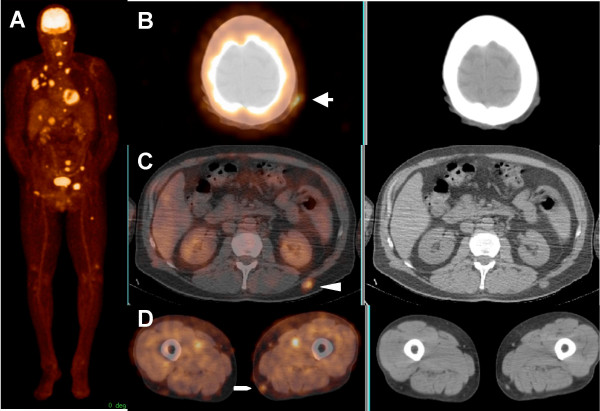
71-year-old male with history of melanoma in the right anterior chest, status post surgical resection and interleukin-2 therapy 2 weeks prior to the PET/CT scan. Maximum intensity projection (MIP) image (A) and transaxial images (B, C and D) show widespread metastatic disease including three ST lesions in the left scalp (arrow), left mid back (arrow head) and left distal thigh (pentagon).
